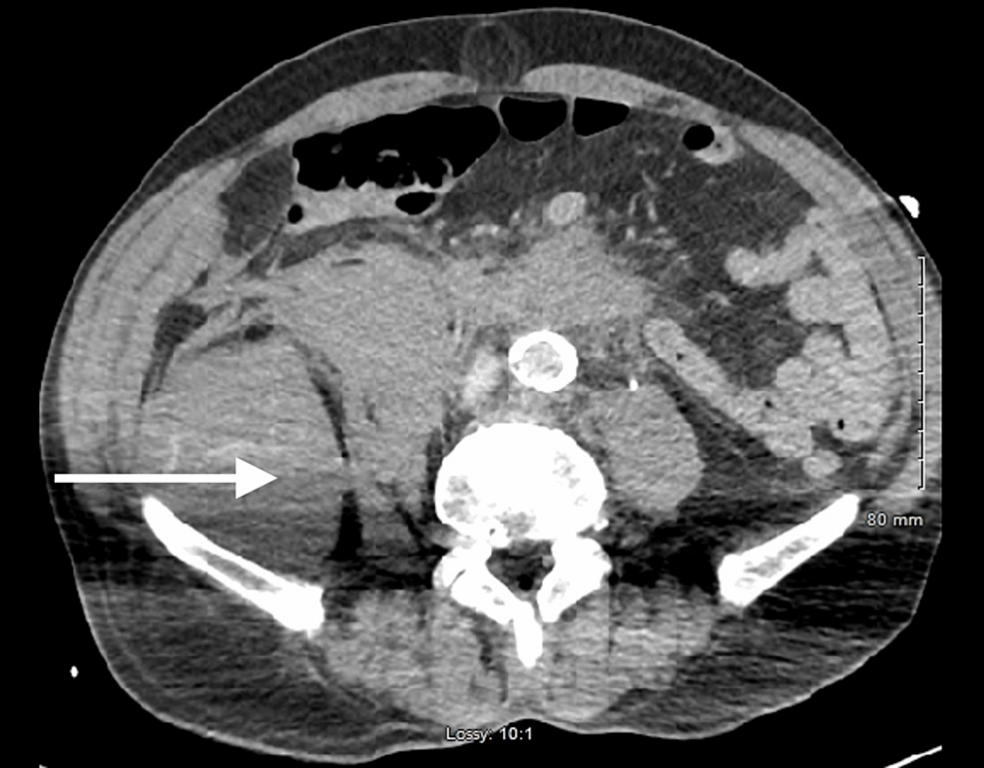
CTA abdomen and pelvis on admission The arrow shows a right iliacus hematoma with contrast extravasation.
